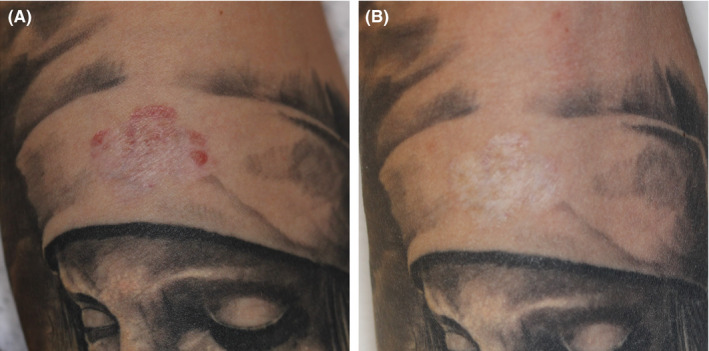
High‐intensity focused ultrasound (HIFU) as back‐up removal of remnant red pigment in a subject (MJ) with tattoo allergy, previously treated with dermatome shaving. A, before HIFU treatment. B, 10 months post‐HIFU; removal was completed and the scar and the esthetic outcome acceptable. Subject was ready for a cover‐up tattoo with a non‐red color. The allergen had been cleared
